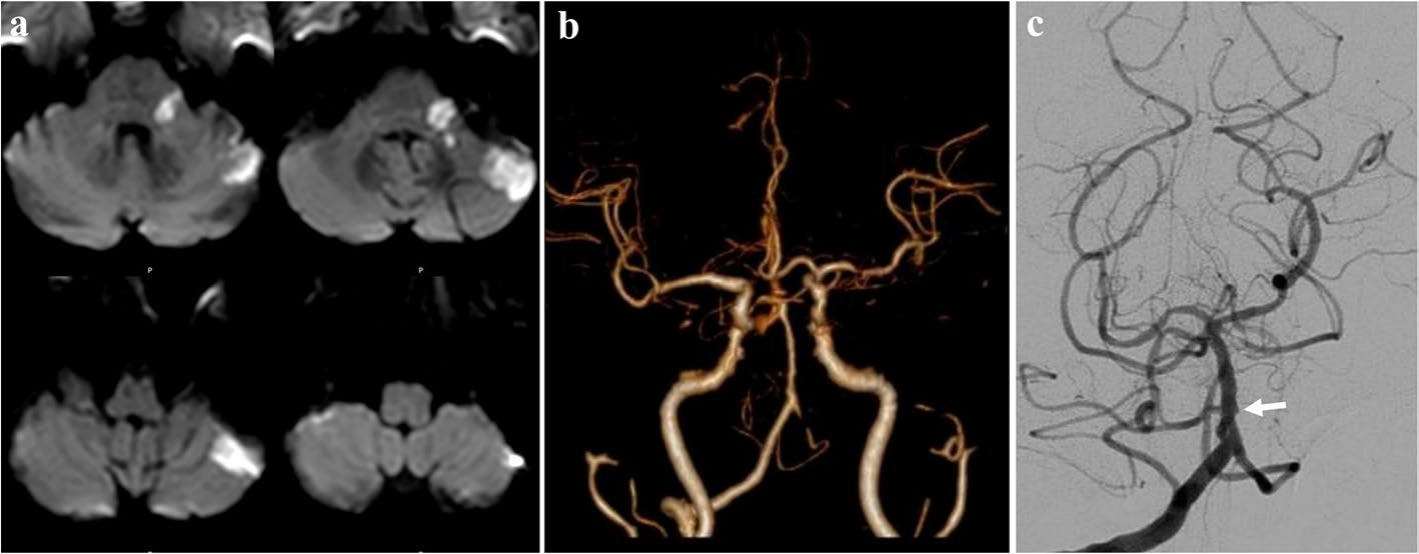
Diffusion-weighted image revealed acute infarction on left brachium pontis and left superior lateral cerebellum (a). Computed tomographic angiography showed the server stenosis and occlusion of left veterbral artery and the absence of bilateral anterior inferior cerebellar artery (AICA) (b). Digital subtraction angiography showed occlusion from left proximal AICA (c)
